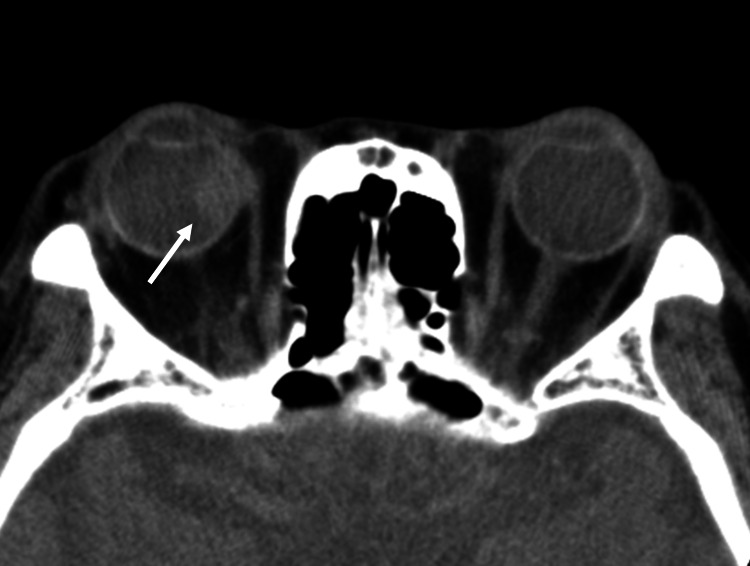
Axial CT scan CT reveals a well-circumscribed ovoid choroidal mass inside the right eyeball, measuring 8 × 12 × 11 mm (arrow). It was iso-attenuated compared to the lateral rectus muscle with infiltration into the internal rectus muscle, which was a suspected extra-scleral extension.
